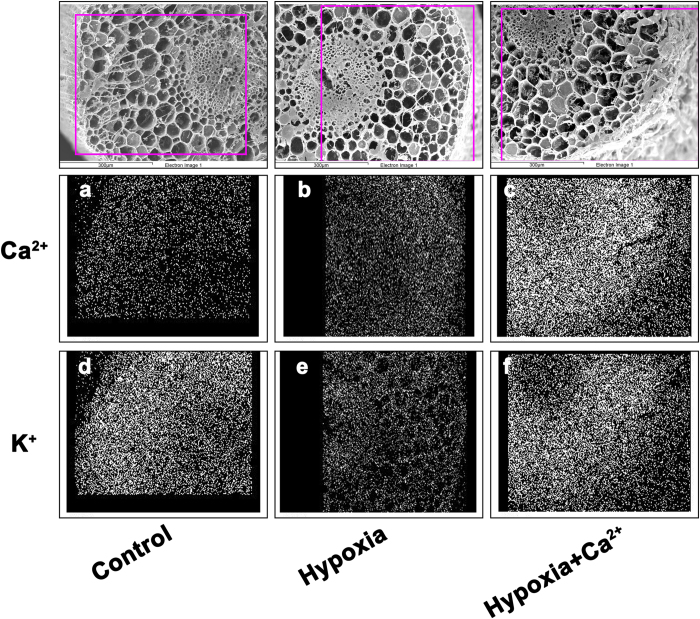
Images of Ca^2+^ and K^+^ distribution in cucumber roots detected using map-scanning of an X-ray. The plants were grown under normoxic conditions (Control), hypoxic conditions (Hypoxia) and hypoxia + 4 mM CaCl_2_ treatment (Hypoxia + Ca^2+^) for 3 d. Samples comprised 1 cm of the root tips. Denser points indicate higher ion concentrations.
